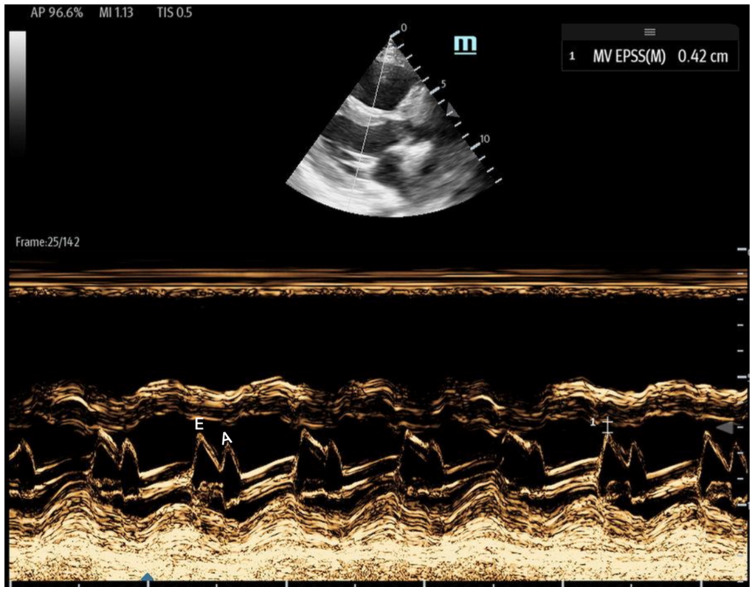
The parasternal long-axis view with the M-mode shows the EPSS measurement, which is the minimal distance between the E wave (initial and maximal opening of the mitral for the passive filling of LV) and the septum. The E wave is followed by the A wave, which is smaller and corresponds to left atrial contraction.
